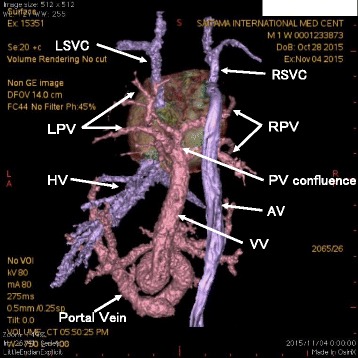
Computed tomography angiography shows that the vertical vein from pulmonary venous confluence goes through the diaphragm and drains into the portal vein, winding its way like a spiral. *LSVC* left superior vena cava, *RSVC* right superior vena cava, *LPV* left pulmonary vein, *RPV* right pulmonary vein, *HV* hepatic vein, *AV* azygous vein, *VV* vertical vein
